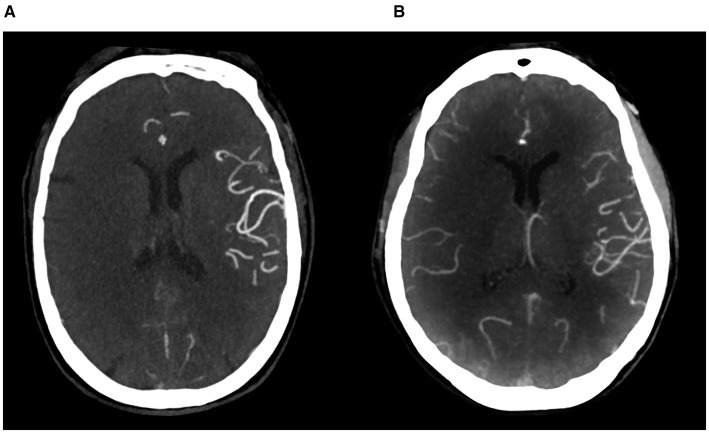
CTA examples of collateral circulation. (A) Collateral grade 0, with absent pial arterial filling in the right anterior circulation. (B) Collateral grade 2, demonstrating ≥50% pial filling of the ischemic right anterior circulation territory. The comparison highlights the striking clinical and perfusion differences between poor and robust collateral networks.
